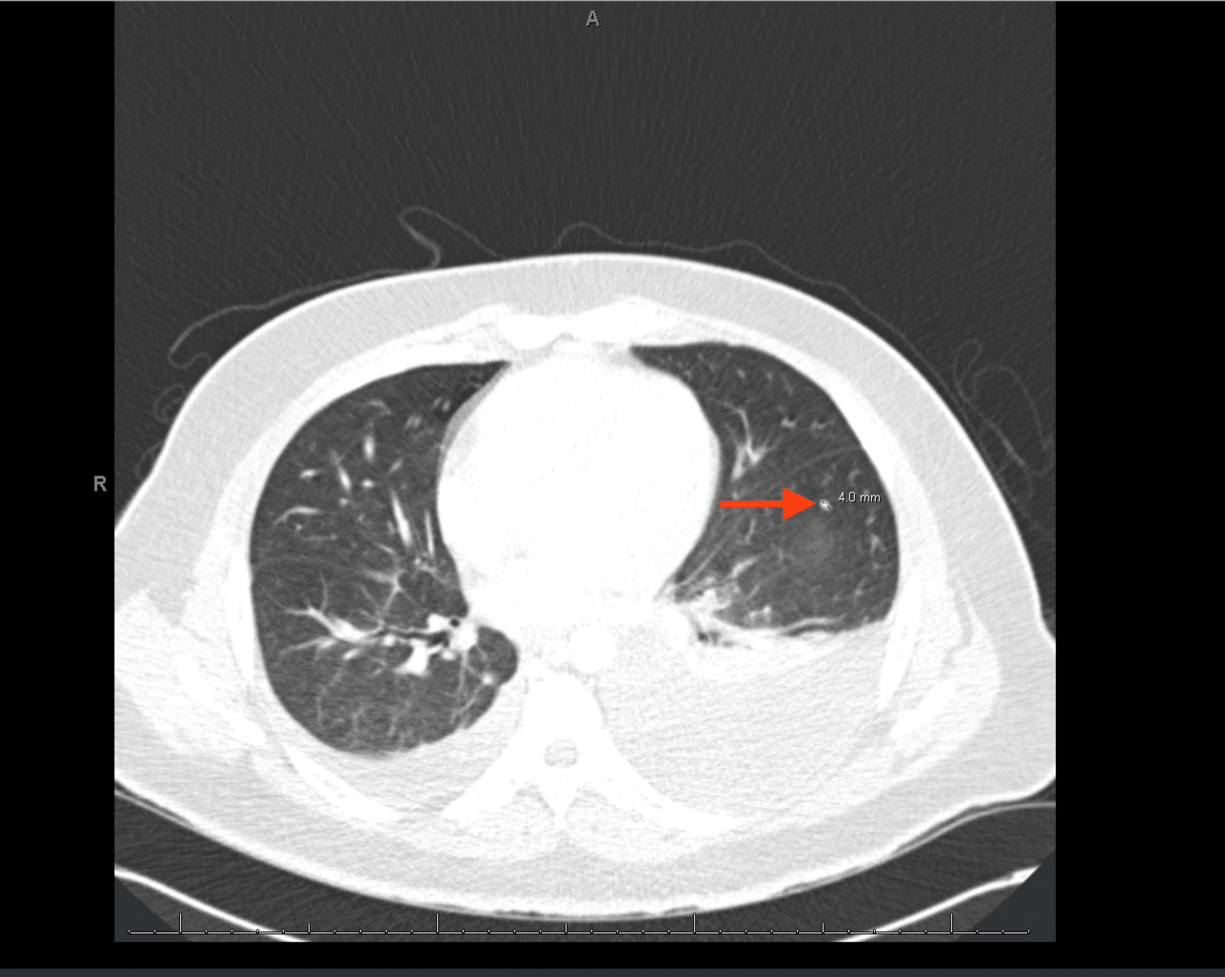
CT scan showing a 4 mm pulmonary nodule. The red arrow indicates a pulmonary nodule on the left lung.
